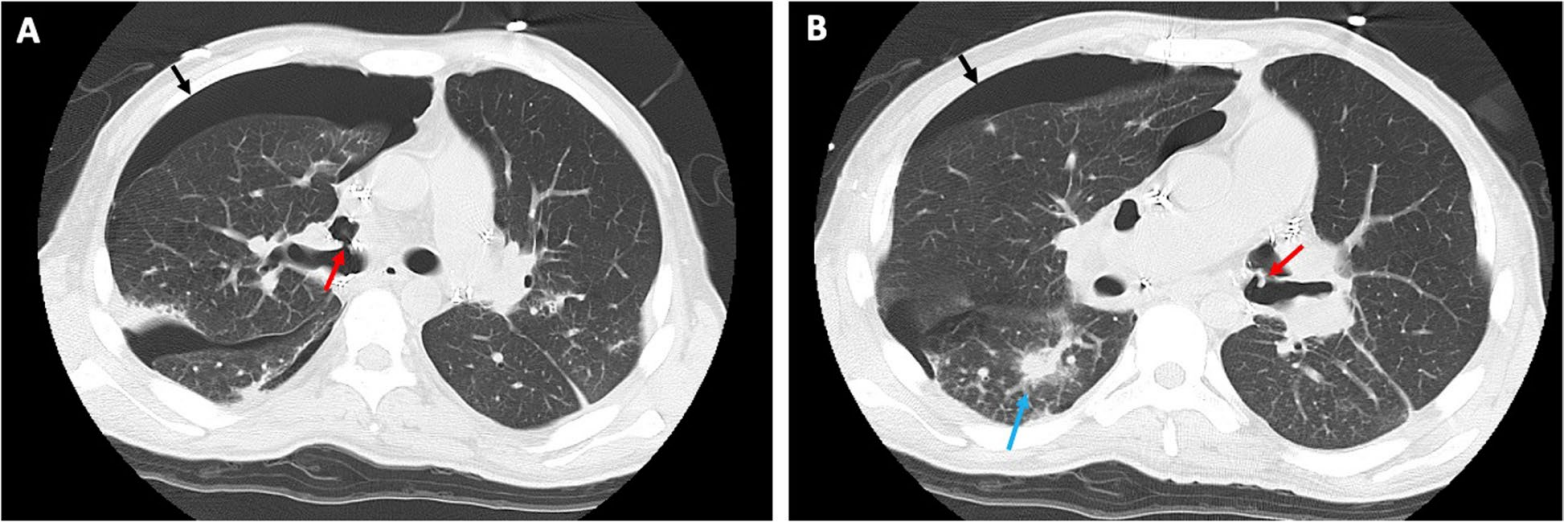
A, B Images of computed tomography scan performed 15 days after the transplantation showing bronchial dehiscence (red arrows), pneumothorax (black arrows), and associated pneumonia (blue arrow)
